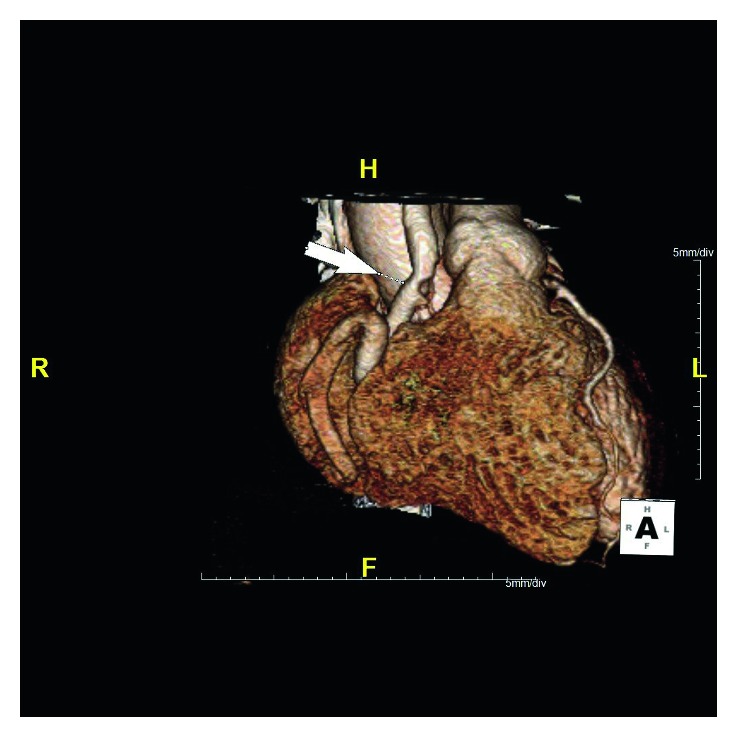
Coronary computed tomography angiogram (volume-rendered image) obtained after her surgery points at the surgically implanted right coronary artery (RCA) in the anterior ascending aorta. There is a filling defect corresponding to a partially occlusive thrombus.
